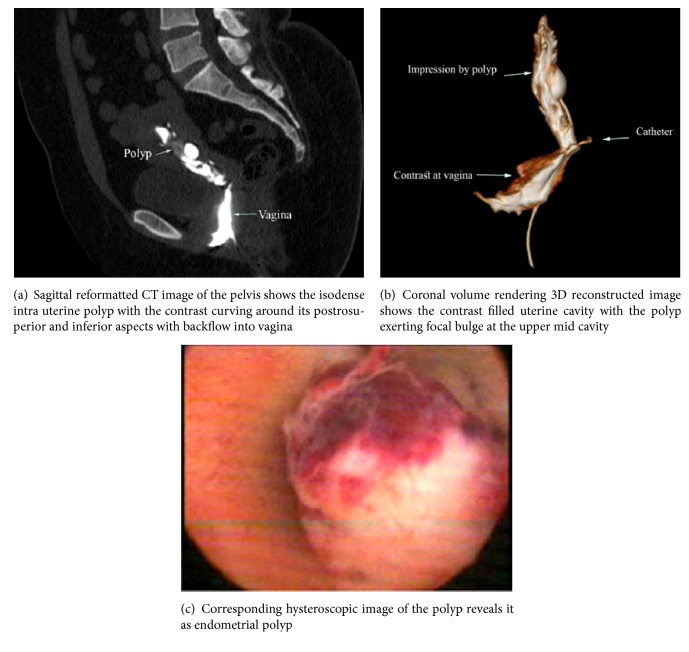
Table 1 tab1: Shows the diagnostic value of CT virtual hysteroscopy in evaluation of uterine cavity abnormalities.
